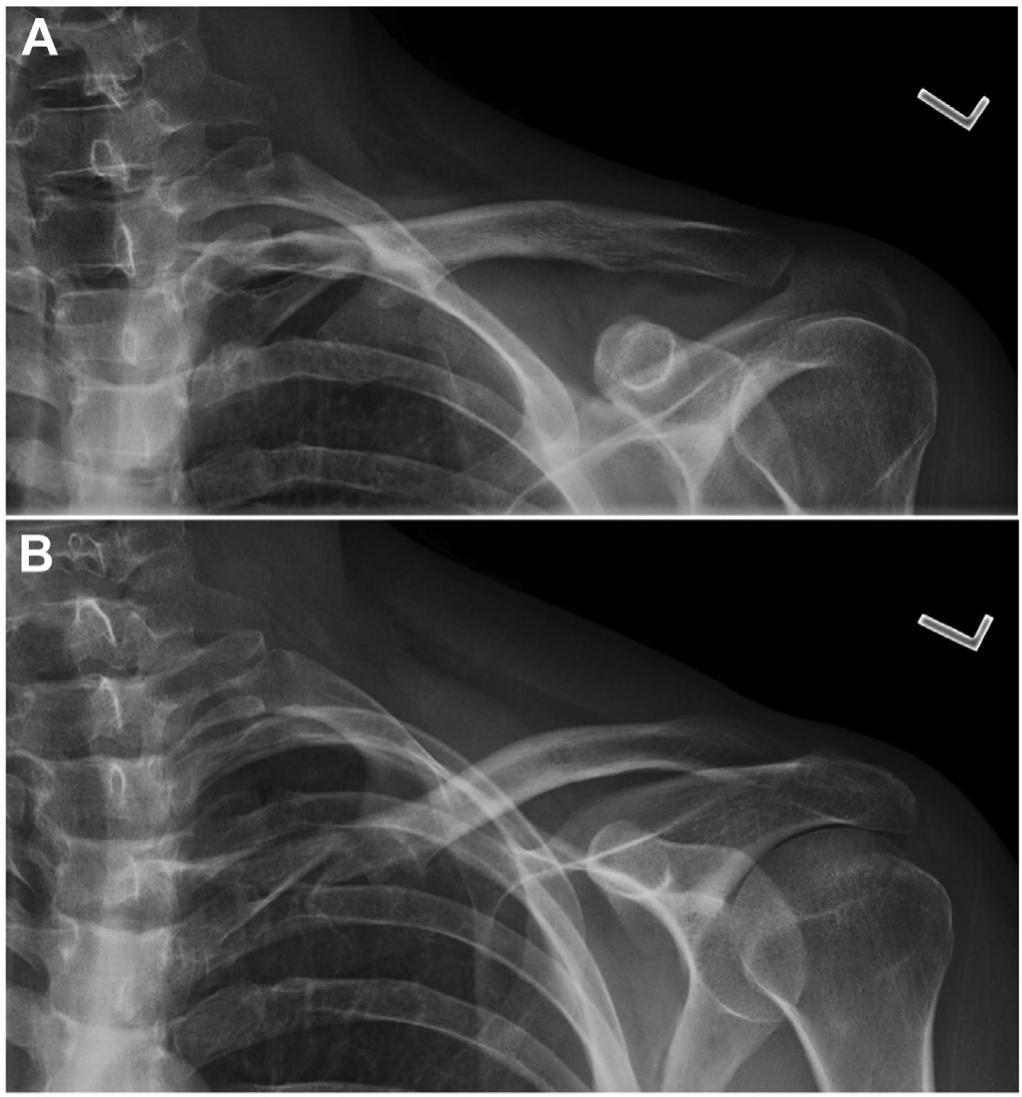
Two preoperative radiographic images, including anteroposterior view of the clavicle (A) and anteroposterior view with cephalic angulation (B) of the left clavicle demonstrate a nonunion of the medial clavicle, obtained at 10 months after initial injury.
